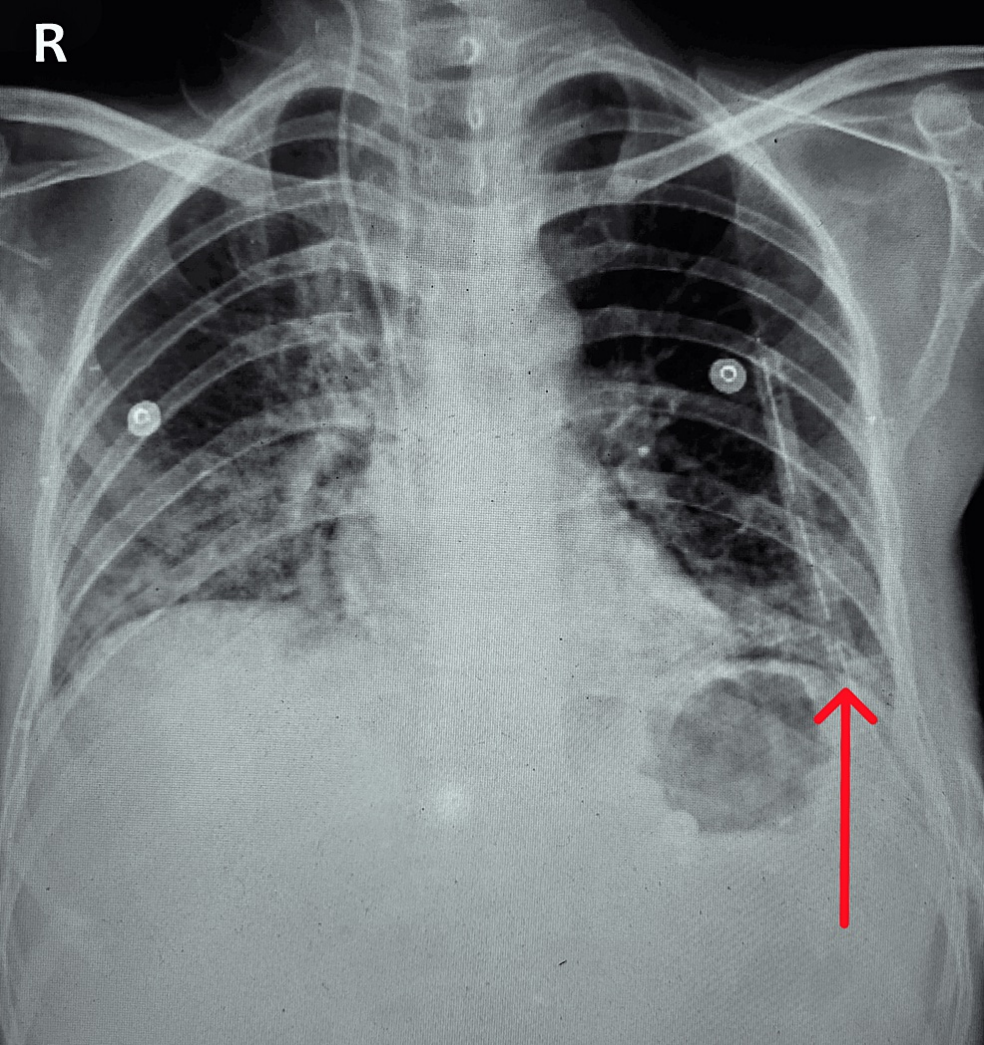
Bilateral lung field haziness on chest x-ray with left pleural effusion and left side ICD in situ
